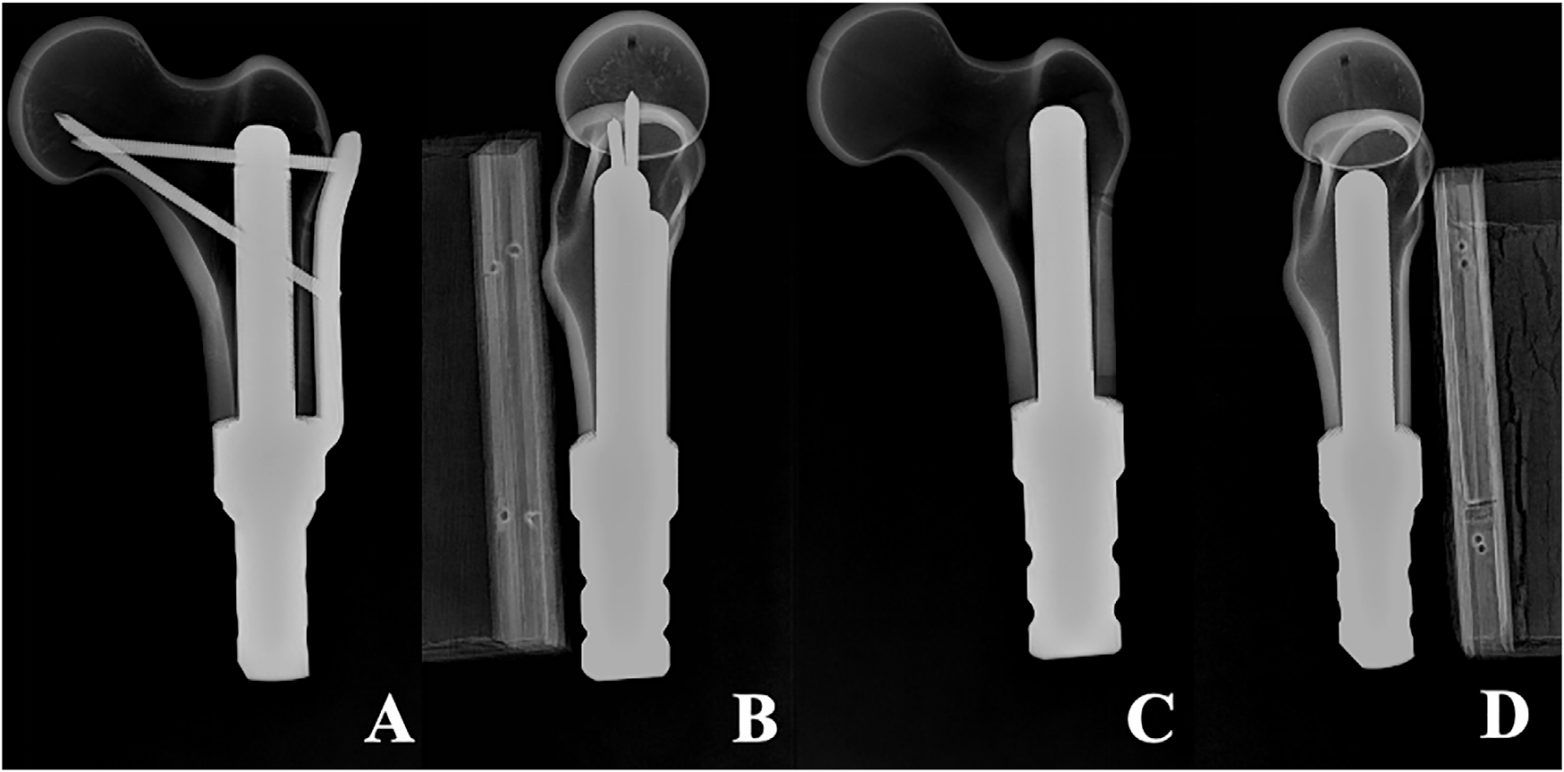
X-ray of two models (A, B) Anterolateral radiograph of Femur-TFS (C, D) Anterolateral radiograph of femur-CS.
